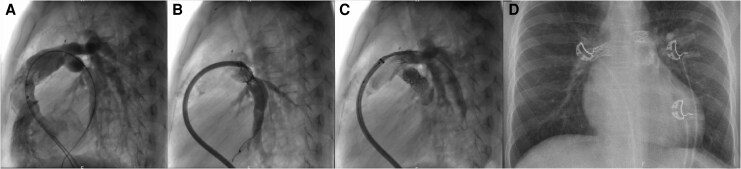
Case 2 angiograms in lateral projection. (*A*) Initial angiogram in the Contegra conduit. The RPA stenosis can be well observed. The LPA is somewhat harder to assess due to overlap. (*B*) Same image as in *Figure 5B* after initial RPA re-stenting before high-pressure balloon angioplasty, in lateral projection. (*C*) Final angiogram in lateral projection confirming resolved bilateral PA stenosis and no obstruction of PA bifurcation by the implanted stents. There is no vascular wall damage. (*D*) Post-procedural chest X-ray confirming unchanged PA stents position and absence of lung parenchymal injury.
